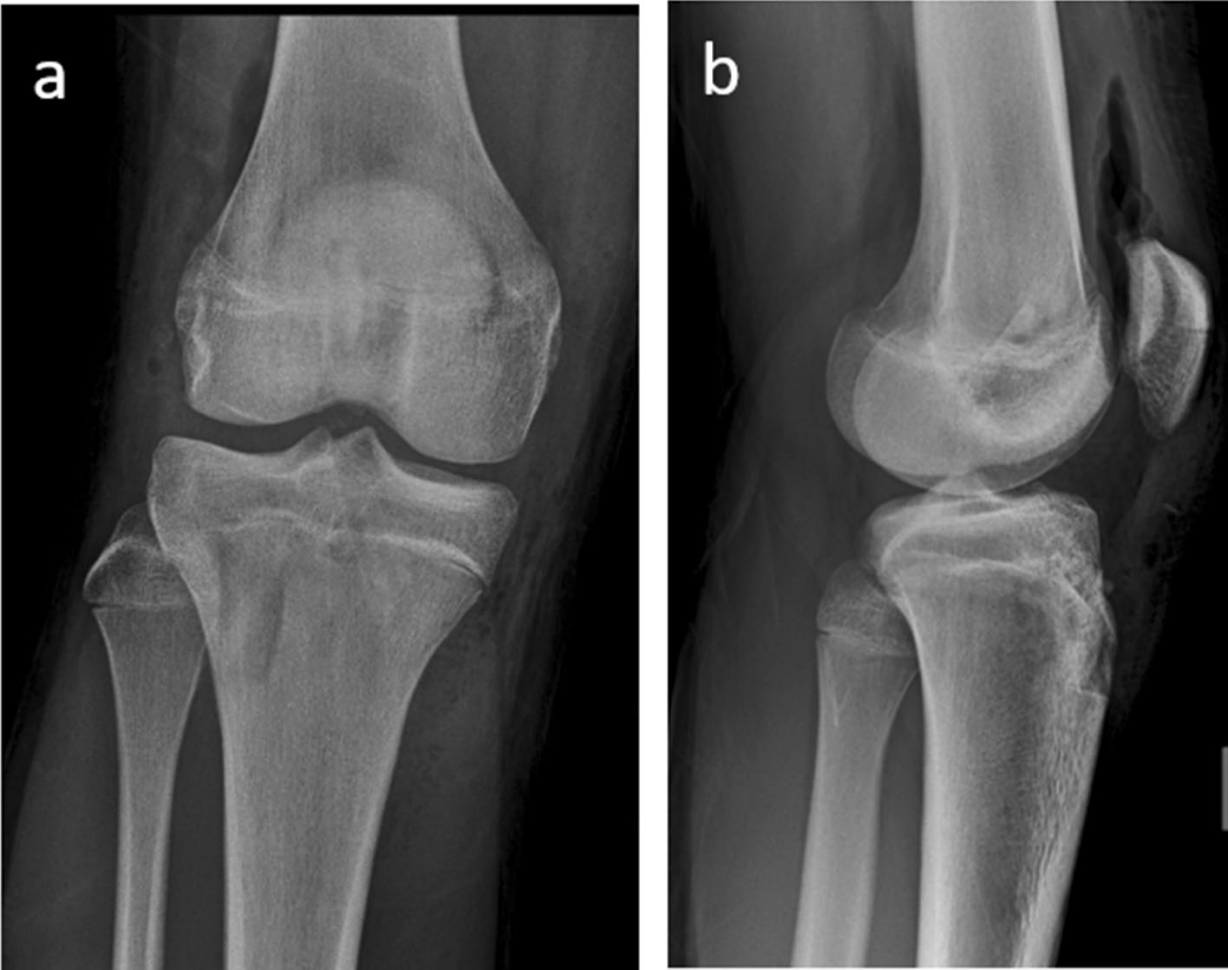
Postoperative X-rays of ACL reconstruction with BPTB in 15-year-old boy male. Anterior–posterior (a) and lateral (b) X-rays of the right knee show open physes and tunnels
